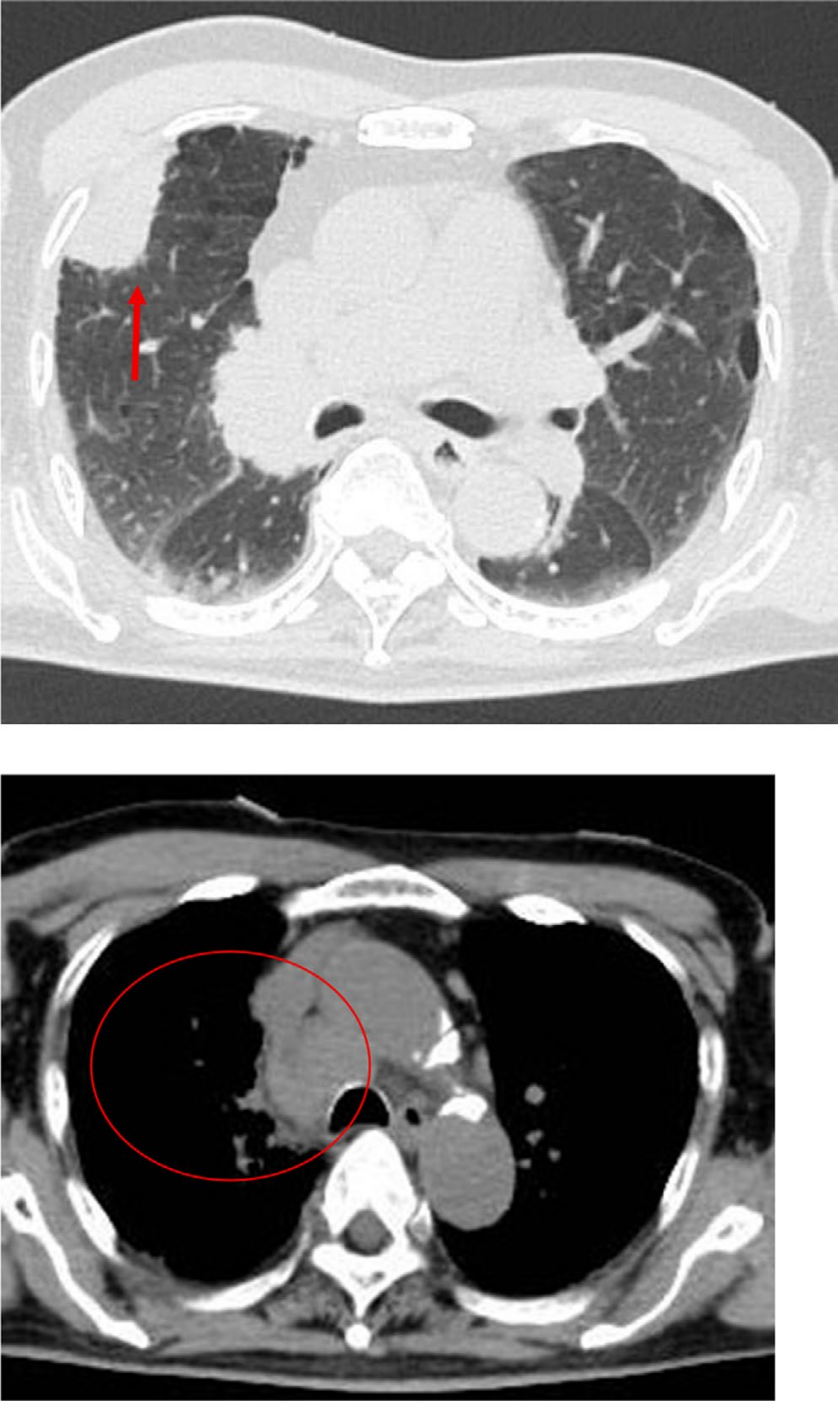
Whole‐body CT on hospital day 1. The red arrow indicates a mass in the right upper lung field. The red circle outlines the area of lymphadenopathy in the mediastinum and right hilar region. The upper and lower images were reconstructed under the lung and mediastinal window settings, respectively. CT, Computed tomography.
